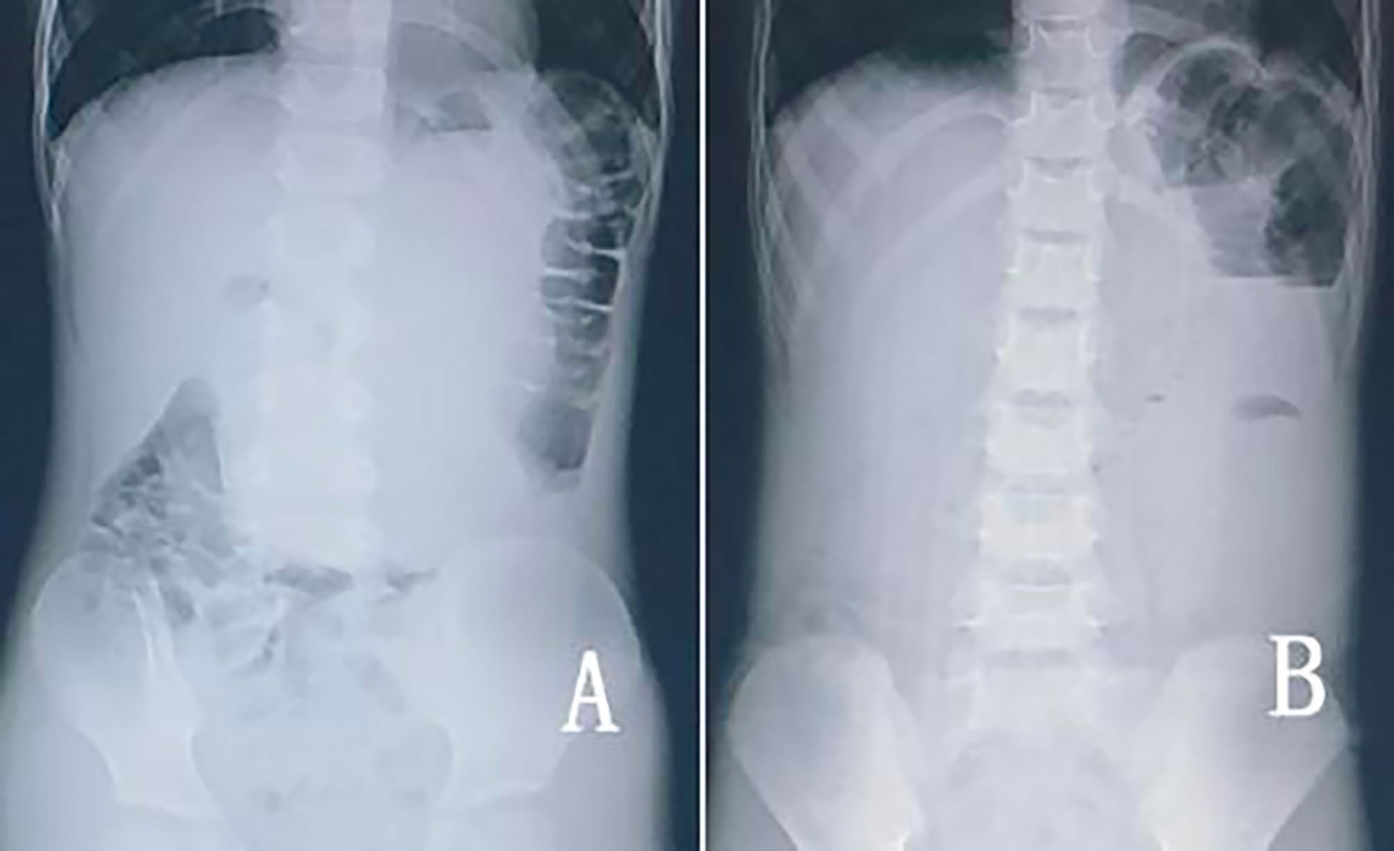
Abdominal radiography: (A) An epigastric mass. (B) Jejunal obstruction
